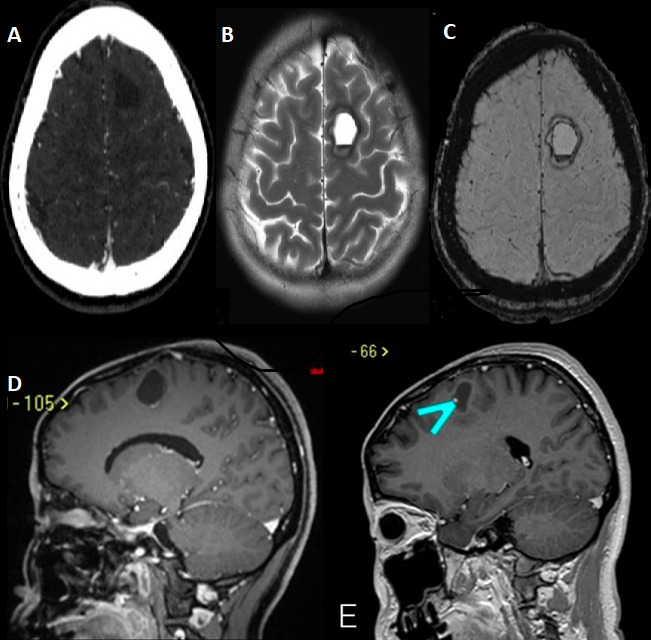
(A) axial CT angiogram shows no vascular malformation; (B) T2 axial image showing a fluid level with small amount of hematocrit level; (C) axial cuts showing SWI sequence shows few punctate foci of blooming artifacts; (D) sagittal section with contrast showing enhancement along inferior aspect of lesion; (E) sagittal cut showing a post contrast enhancement of a nodule (*blue arrow*)
